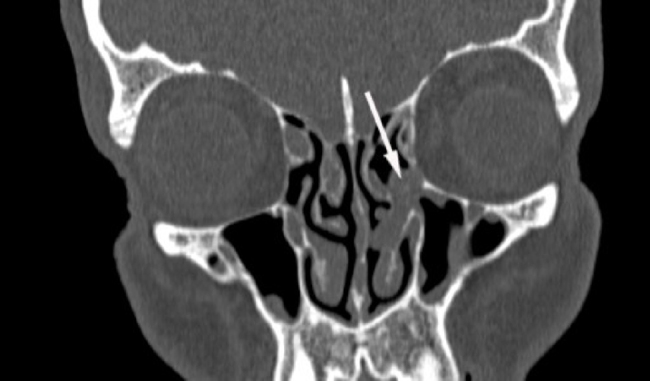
CT scan of the sinuses. A polyp obstructing osteomeatal complex (white arrow) in a patient diagnosed with acute myeloid leukemia, qualified for allogeneic hematopoietic stem cell transplantation.
